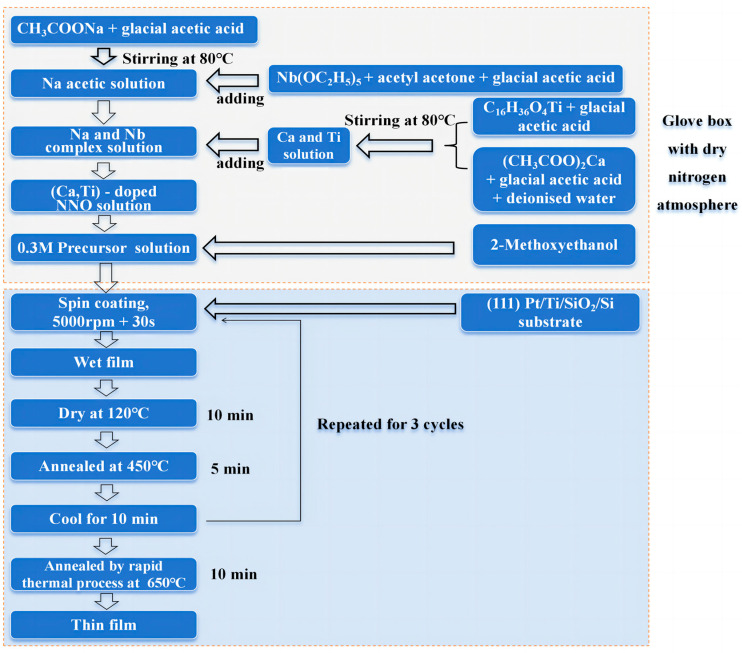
Preparation process for (1−*x*)NN-*x*CT thin films by sol-gel method.
